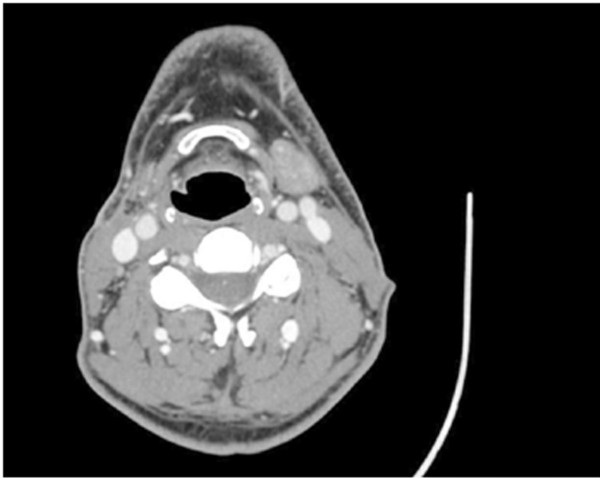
Axial CT scan showing the involvement of the right parotid region.
